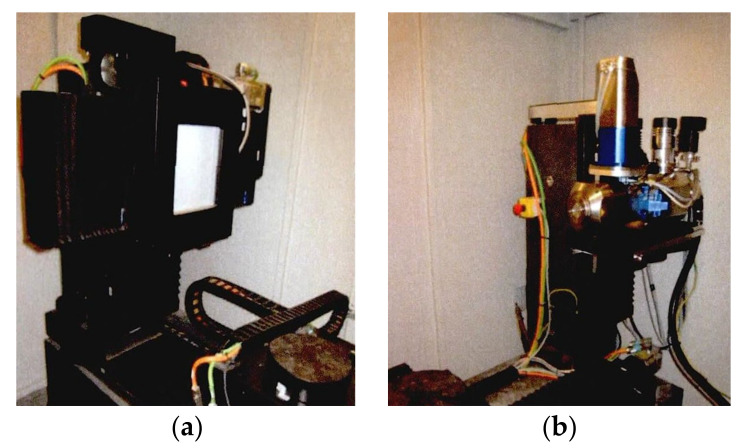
CT scanning system: (a) flat panel detector, (b) X-ray source.
